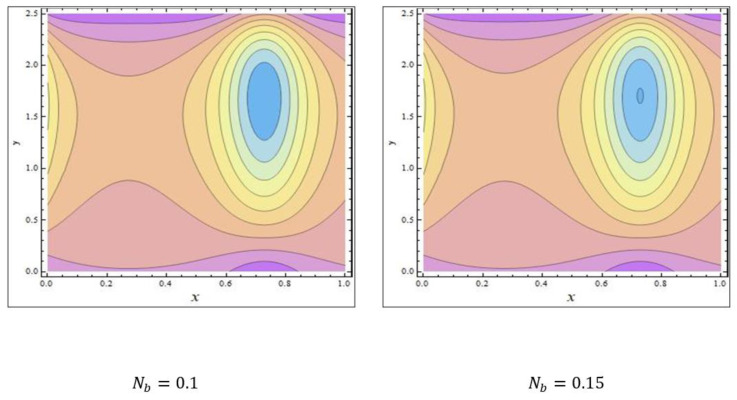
The streamlines of $N_{b}$. The values of the other parameters were $N_{CT}=0.3,\;N_{TC}=0.7,\;G_{rc}=0.4,\;G_{rF}=0.1,\;G_{rt}=0.5,\;N_{t}=0.7,\;M=3.5,\;\phi =\frac{\pi }{6},\Gamma =0.8,\;\beta =0.7,\;m=0.6,\;Q=2.37$.
